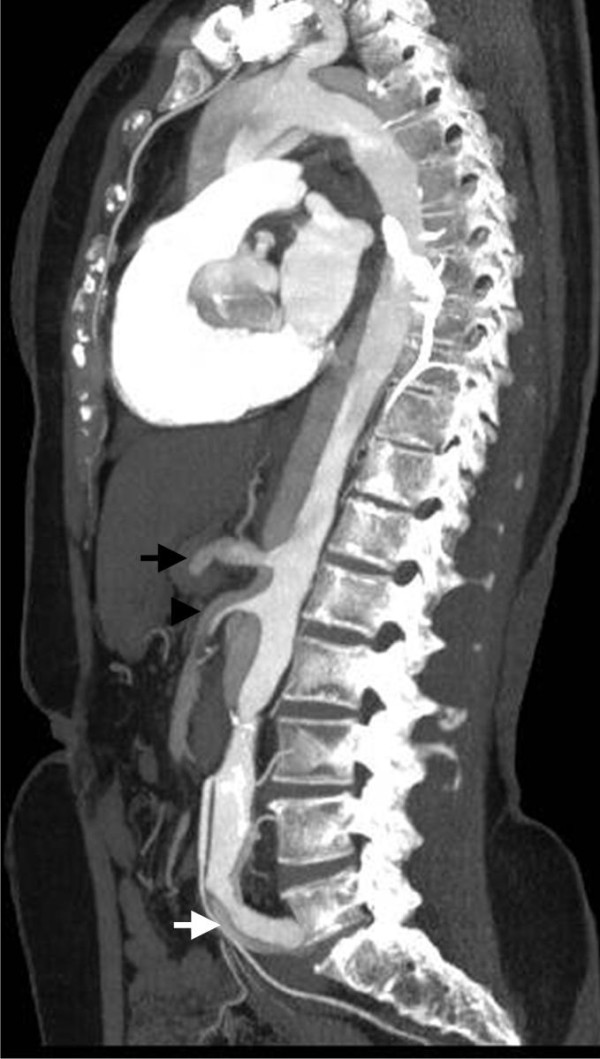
Multidetector Computed Tomography Angiography (MDCTA): Median plane depicts the extended dissection from the ascending aorta, passing through the origins of celiac trunk (black arrow) and superior mesenteric artery (arrow head) down to the iliac arteries (white arrow).
